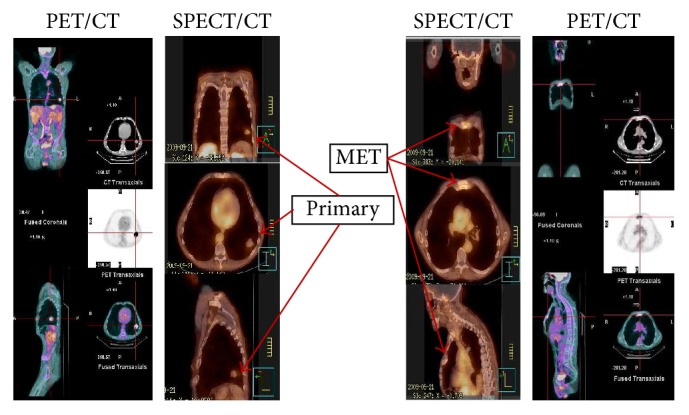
Comparative SPECT/CT: ^99m^Tc- EC-G and PET/CT: ^18^F- FDG image sets on a 56-year-old patient having a biopsy confirmed primary NSCLC and diagnostic CT contrast confirmed metastasis to the sternum.
